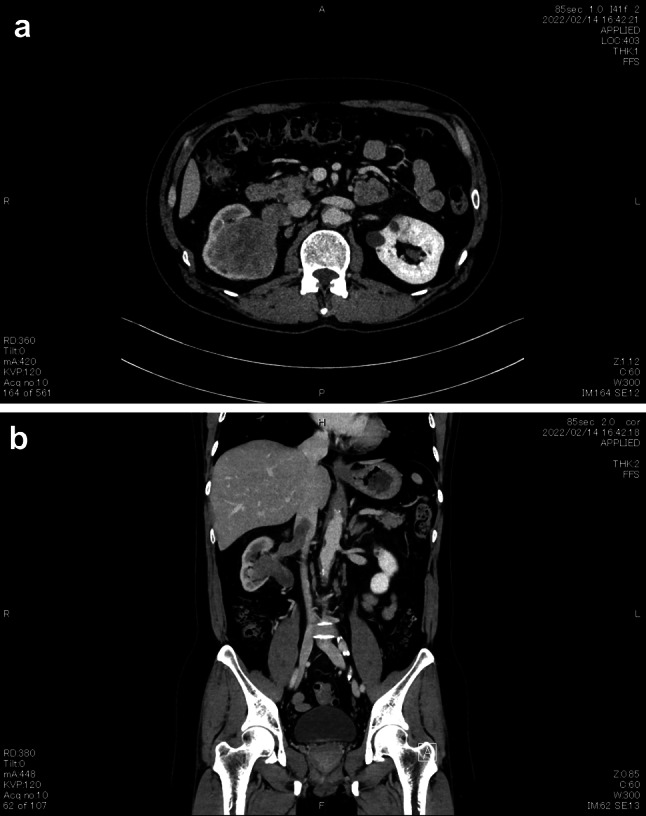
CT images of the right renal tumor on presentation. a CT image showing a 75- × 60-mm right renal tumor. b Contrast-enhanced CT image showing thrombus within the renal vein and a 35-mm-long thrombus in the inferior vena cava
